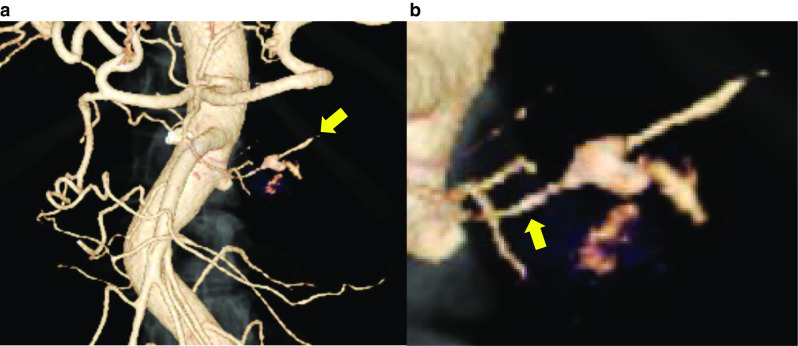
Three-dimensional computed tomography angiography showing a transverse pancreatic artery aneurysm from the superior mesenteric artery (SMA). a Celiac artery and SMA branching from the aorta. An aneurysm (yellow arrow) is observed on the left side of the SMA. b An enlarged image of the aneurysm. It branched from the SMA (yellow arrow)
